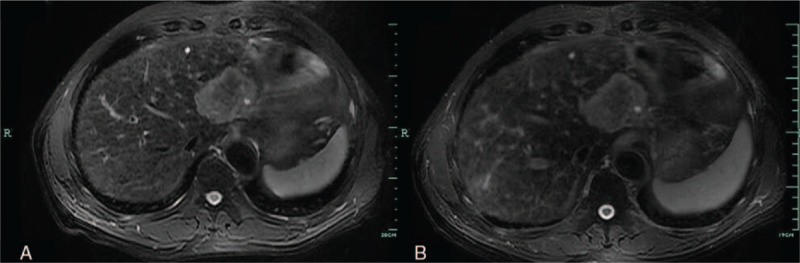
Comparison of magnetic resonance imaging obtained on admission (A) and after 2 months (B).
